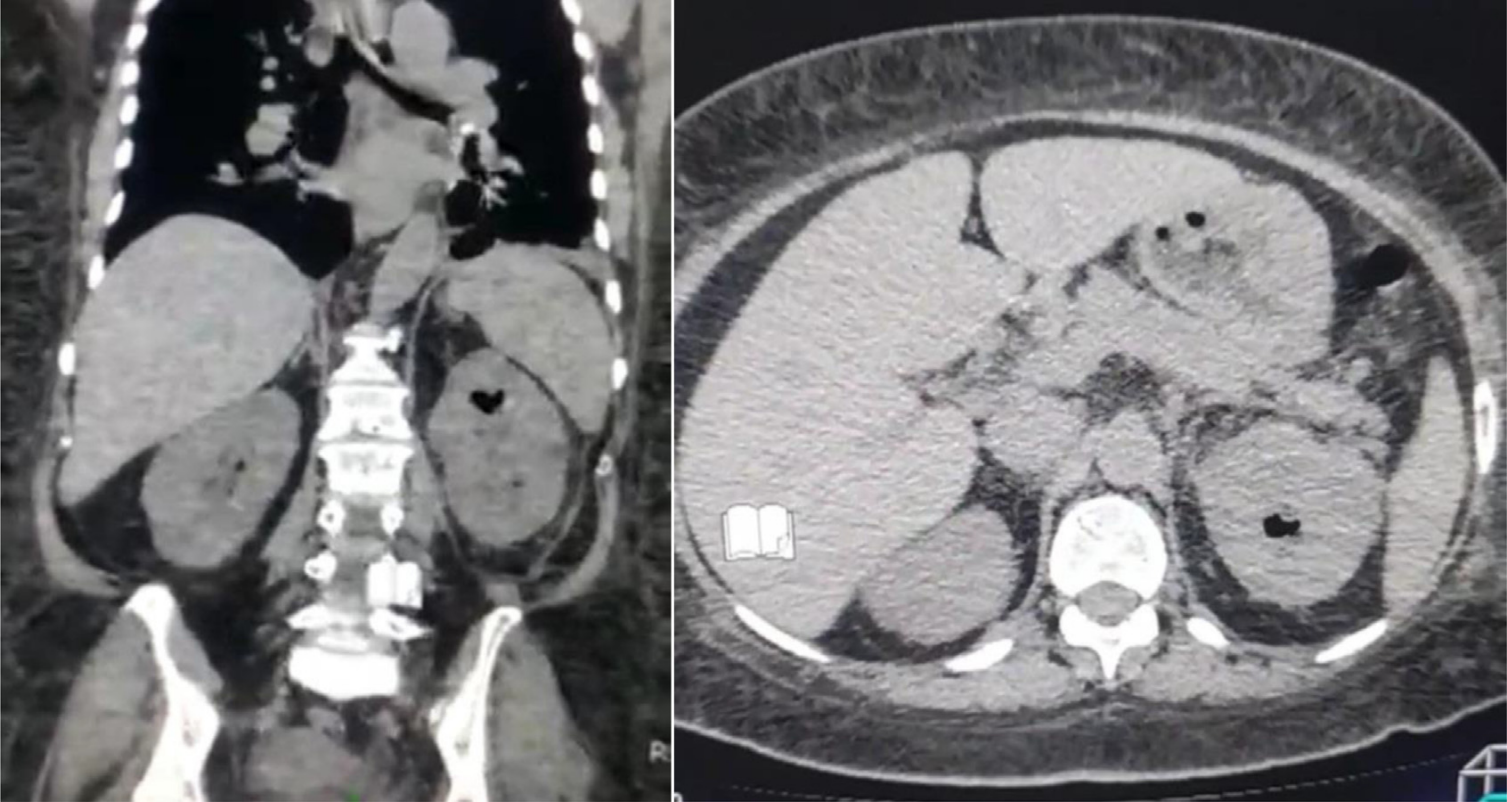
Computed tomography (coronal and axial views) showing gas in the left kidney.
